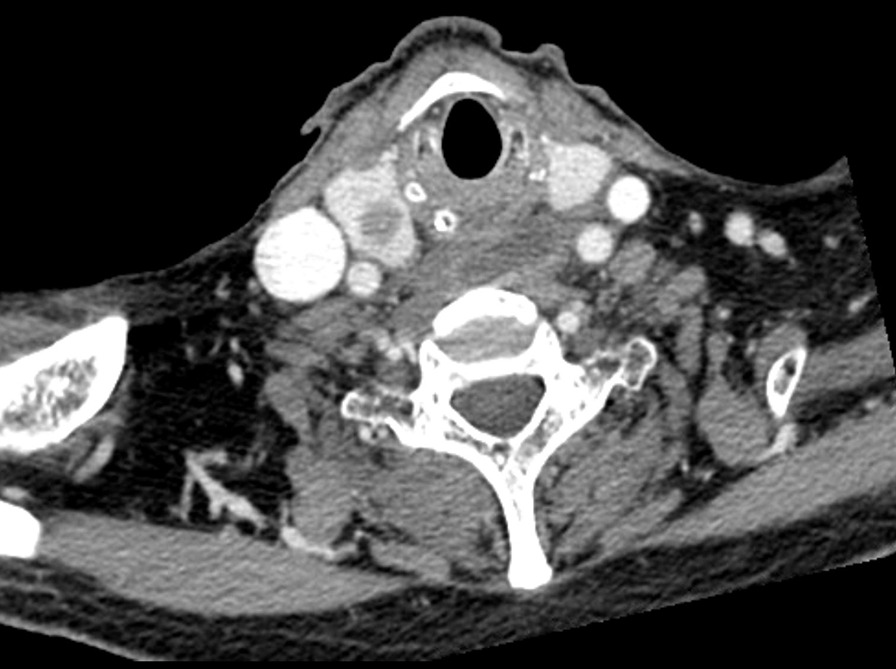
Follow-up CT taken three weeks after transcatheter arterial embolization reveals a low-density lesion indicating an old hematoma in the posterior neck space
